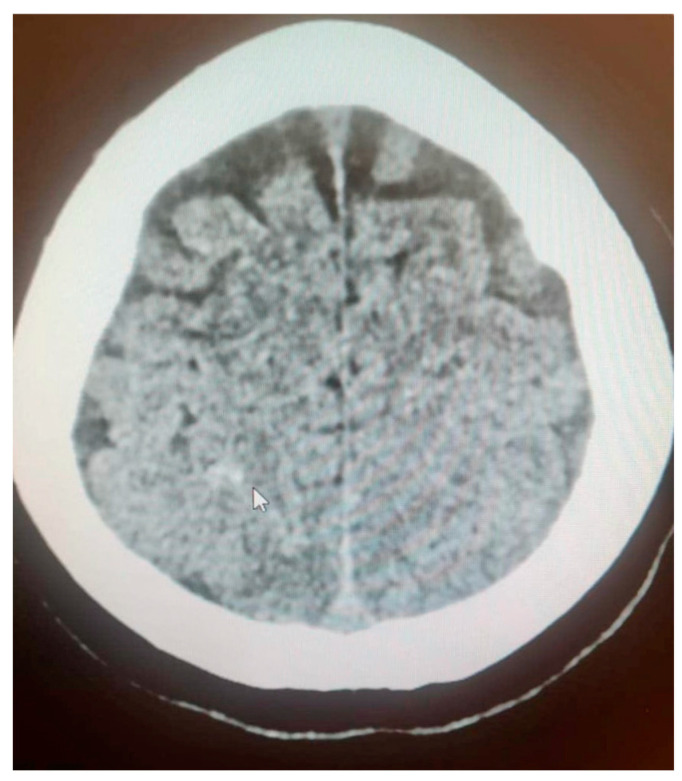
Brain CT confirmed cerebral edema, areas of hypodensity and SAH (white arrow).
